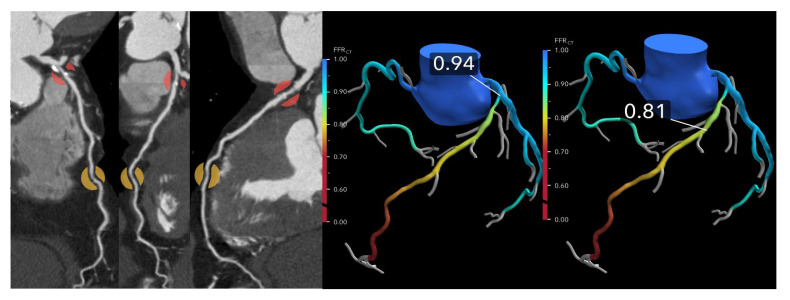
Lesion-specific ischemia: (Left): A curved multiplanar reformatted coronary CTA image in a 60-year-old man reveals moderate stenosis in the anterior left anterior descending (LAD) artery. (Right): The corresponding FFR-CT image shows an FFRCT value of 0.81 measured 2 cm distal to the lesion. The difference between the proximal and distal FFR-CT values across the lesion is 0.13, which exceeds the abnormal threshold of 0.12, confirming hemodynamically significant ischemia.
